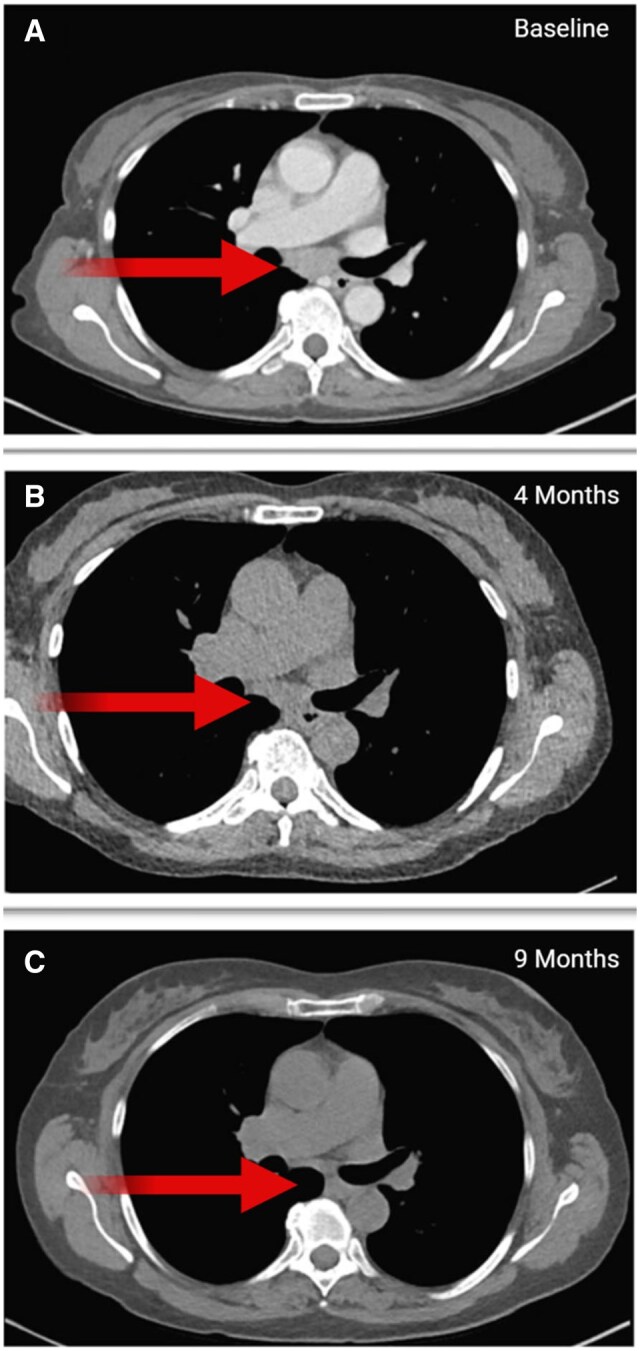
Vorasidenib radiological response in the subcarinal lymph node. Radiographic response to vorasidenib therapy. (A) Baseline CT chest before the initiation of vorasidenib demonstrating progressive mediastinal lymphadenopathy. (B) CT chest obtained 4 months after the initiation of vorasidenib demonstrating interval decrease in mediastinal lymphadenopathy. (C) CT chest obtained 9 months after the initiation of vorasidenib demonstrating sustained stability of the subcarinal lymph node and overall mediastinal disease burden.
